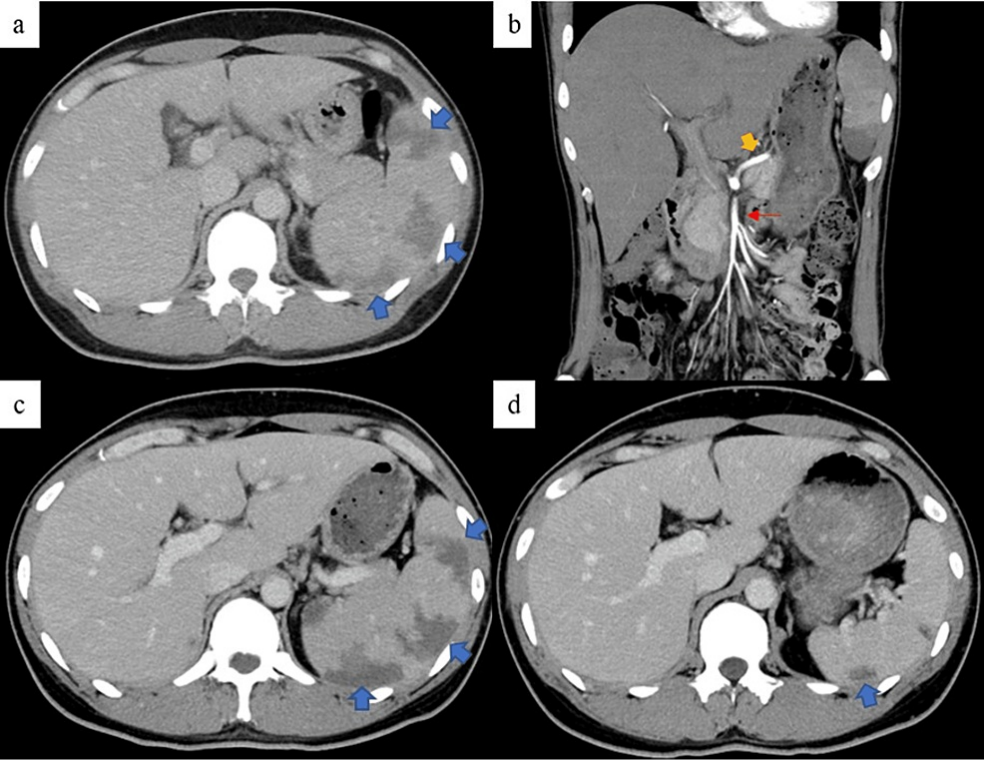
Abdominopelvic computed tomography image with the splenic infarction indicated by the blue arrows. (a) is an axial image at admission. Splenomegaly with localized splenic infarction can be observed. The splenic volume was 526 mL. (b) is a coronal image at admission. The splenic artery (SpA; orange arrow) branches from the superior mesenteric artery (red arrow). The maximum diameter of the SpA was 4.9 mm. (c) is an axial image obtained on the fourth day of hospitalization. Splenomegaly remains unchanged, and there is no enlargement of the infarcted area. (d) is an axial image obtained two weeks after discharge. Splenomegaly has improved markedly, and the infarcted area has shrunk. The splenic volume was 228 mL.
